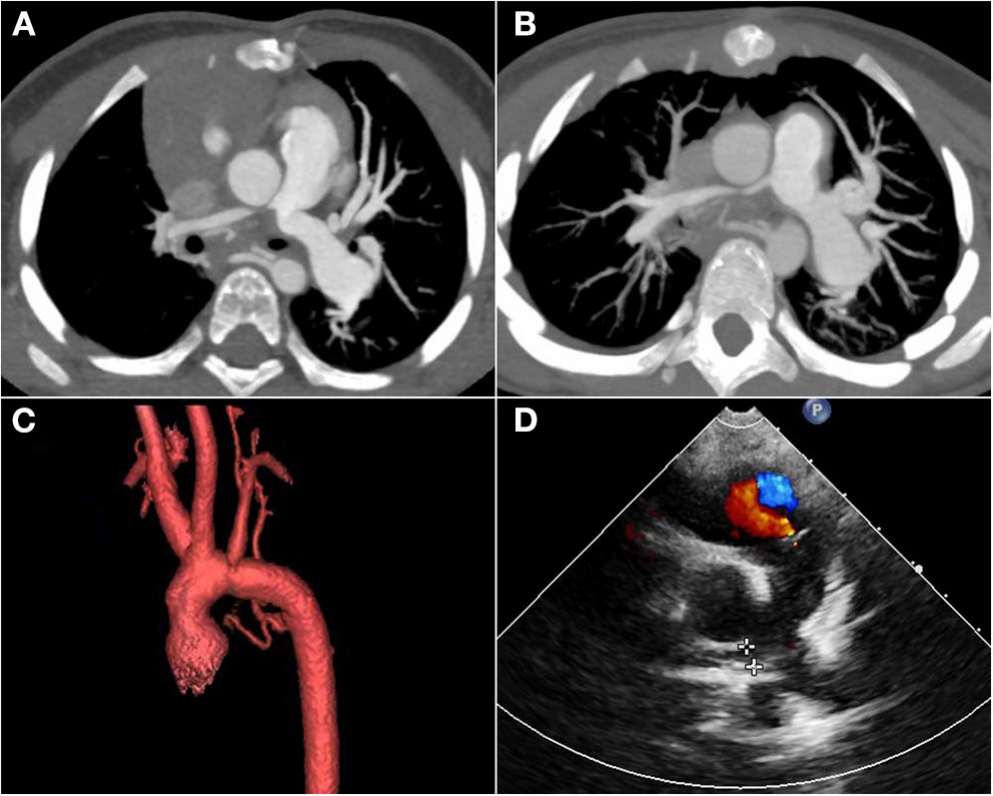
Post-operative follow-up images of patient No. 2. (A) CT images showed that ascending aorta oppressed the opening of RPA and LPA 12 months after the primary operation. (B) CT images showed RPA stenosis again 6 months after the first re-operation. (C) The post-operative three-dimensional imaging of the aortic arch showed that the aortic arch and its branches were in good shape. (D) The latest ultrasound images showed good development of RPA with a gradient of 9 mmHg. RPA, right pulmonary artery; LPA, left pulmonary artery.
